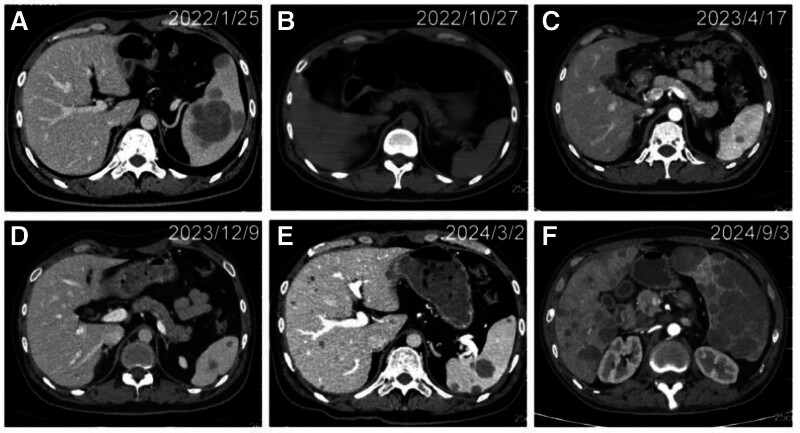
CT evaluation of hepatic and splenic metastases throughout treatment. Time points for each panel: (A ) January 25, 2022 (baseline, pre-treatment); (B) October 27, 2022 (9-month follow-up); (C) April 17, 2023 (15-month follow-up); (D) December 9, 2023 (22-month follow-up); (E) March 2, 2024 (25-month follow-up); (F) September 3, 2024 (31-month follow-up). CT = computed tomography.
